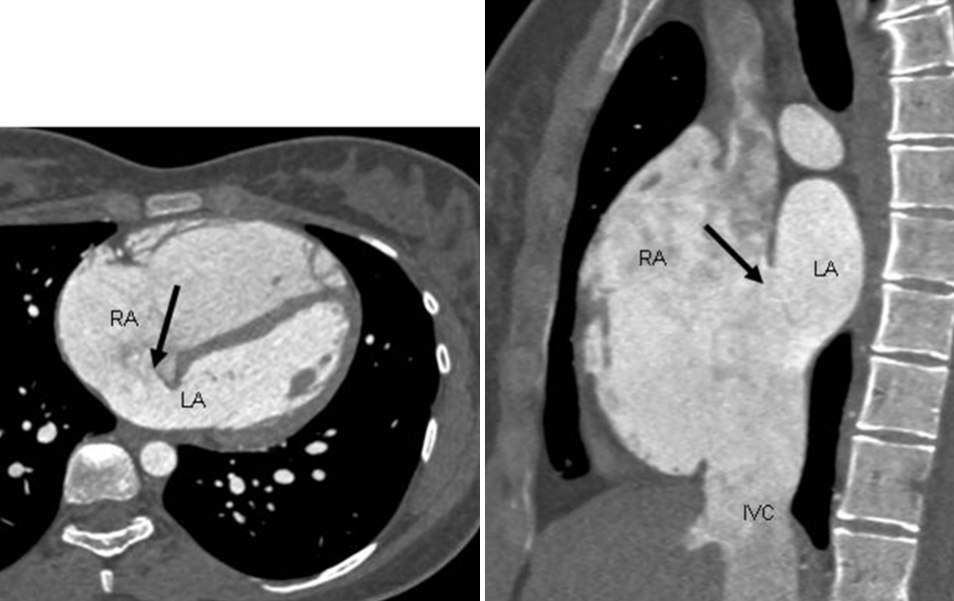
An inferior sinus venosus atrial septal defect (ASD). A young male patient underwent preoperative multidetector computed tomography angiography, which revealed a large multifenestrated inferior sinus venosus ASD in axial (a) and sagittal (b) images. RA = right atrium, LA = left atrium, IVC = inferior vena cava.
